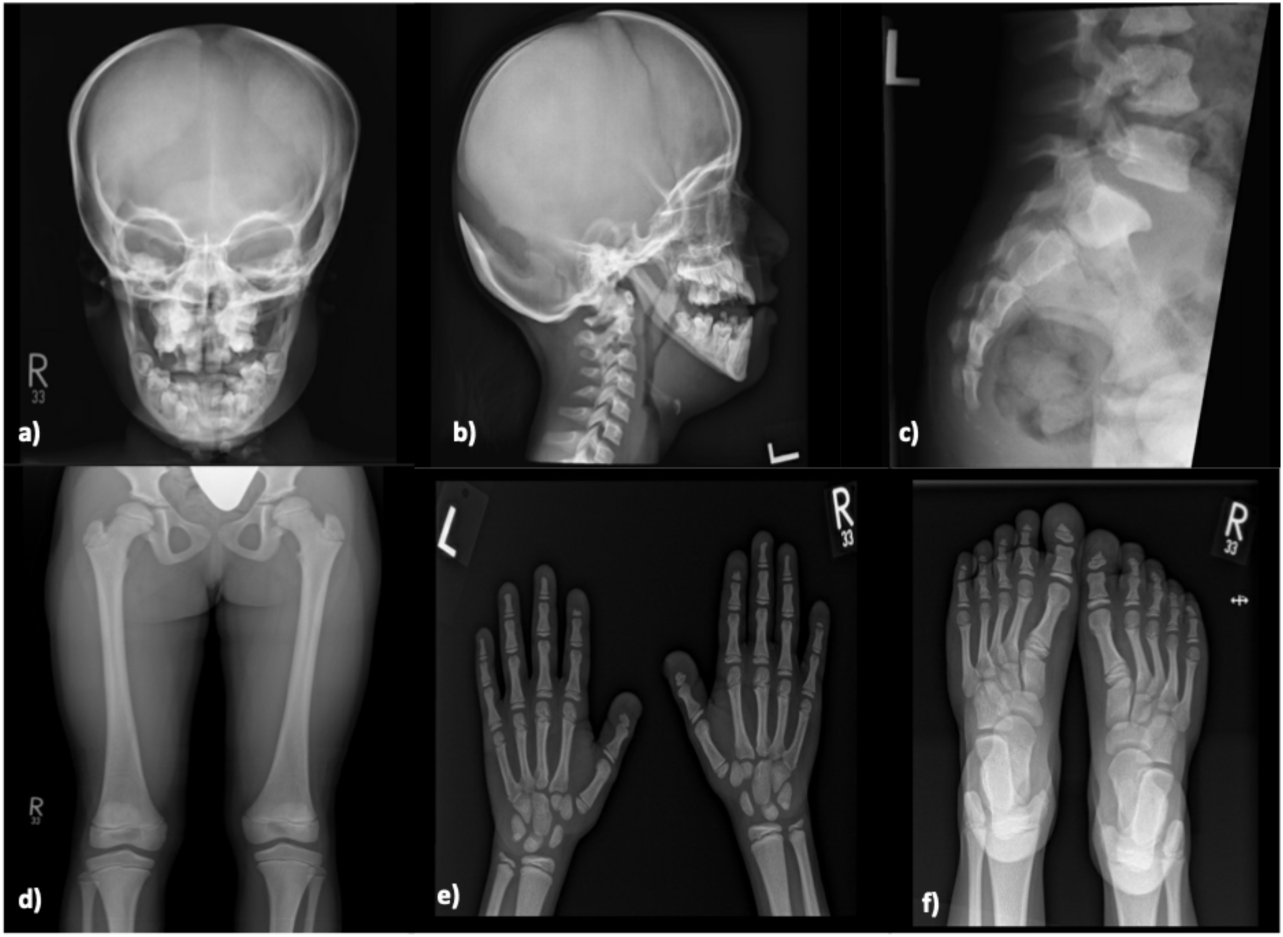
Skeletal X-ray of a male patient done at 11-year-old (Case 6) revealing moderate diffuse increased density of the skeletal bones with persistent open skull sutures, and widenings most prominent of the posterior sagittal and lambdoid sutures, anterior fontanelle, and straight mandible angles with abnormal teeth are noted (a, b). Irregularity at the posterior elements of the C2, C3 are seen (b). Spondylolysis /grade 1 spondylolisthesis of L5-S1 (c). Mild to moderately hypoplastic bilateral acetabulae are noted, with 8 mm lateral uncoverage of the right femur head, 12 mm lateral uncoverage of the left femur head seen (d). Prominent tufts resorptions /distal acro-osteolysis of bilateral feet and hands distal phalanges are noted (e, f). Overall Sclerosis and dysplastic skeletal features are compatible with Pycnodysostosis.
